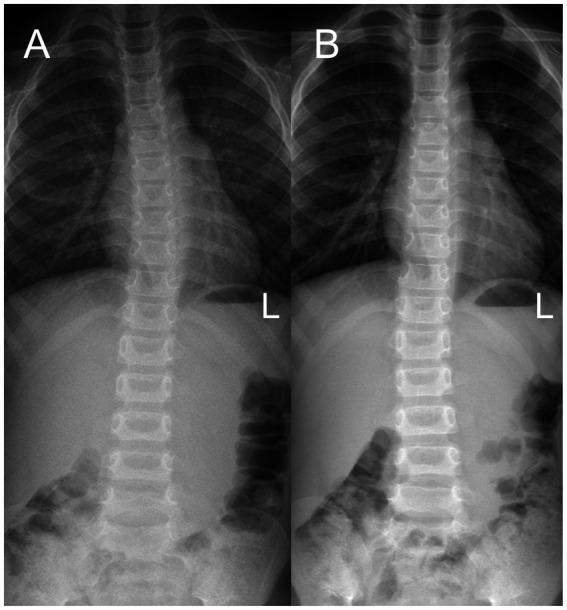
Full-length spinal anteroposterior X-ray radiography in case 1. (A) The Cobb angle was 10° before treatment. (B) The Cobb angle was 3° after 3 months of MSIT treatment.
